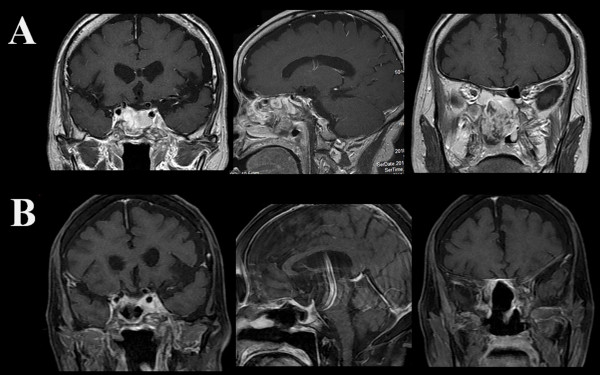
Recurrence of the tumor. A. Four months after the first surgery, MRI demonstrates rapid regrowth of the tumor filling sphenoid and ethmoid sinuses and nasal cavity. B. A residual tumor is seen in the cavernous sinuses after massive tumor resection.
